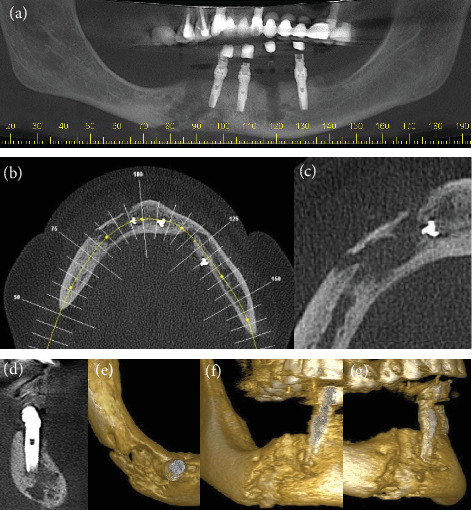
(a–g) CBCT scans show the osteolytic and radiolucent area with cortical destruction of the buccal plate and base of the right quadrant of the mandible. (e–g) 3D reconstruction shows bone destruction.
